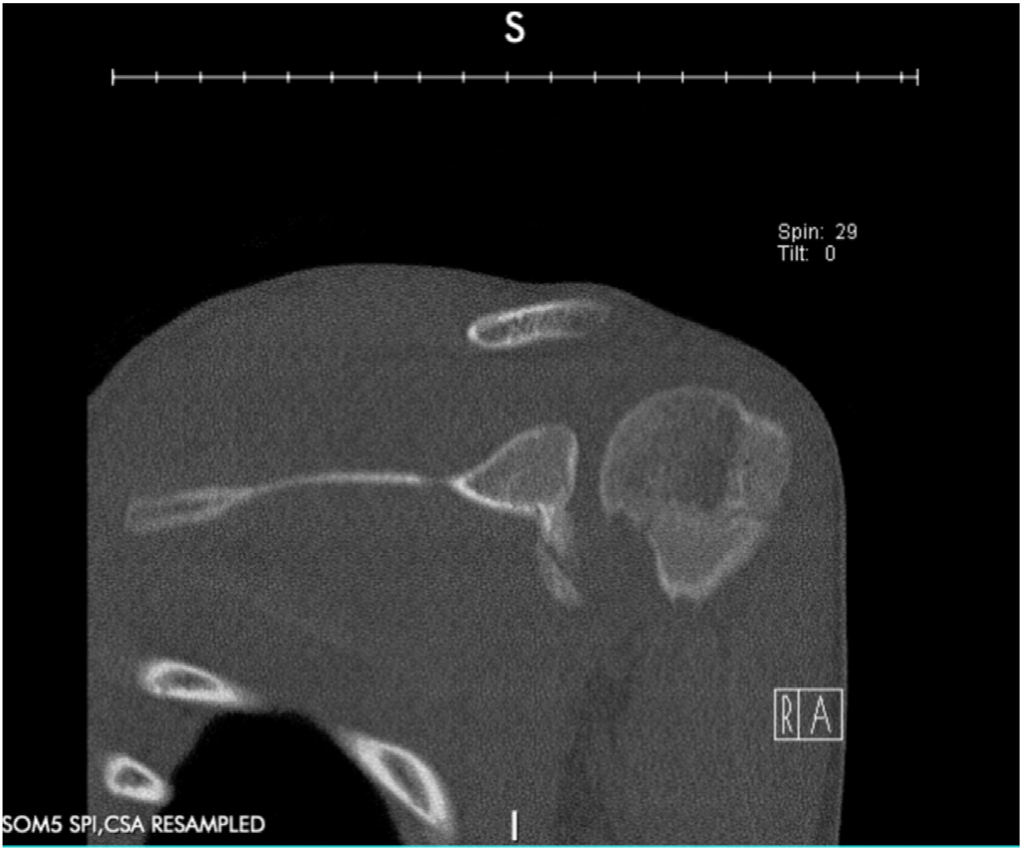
Coronal CT image taken after anterior dislocation revealing bony Bankart lesion.
